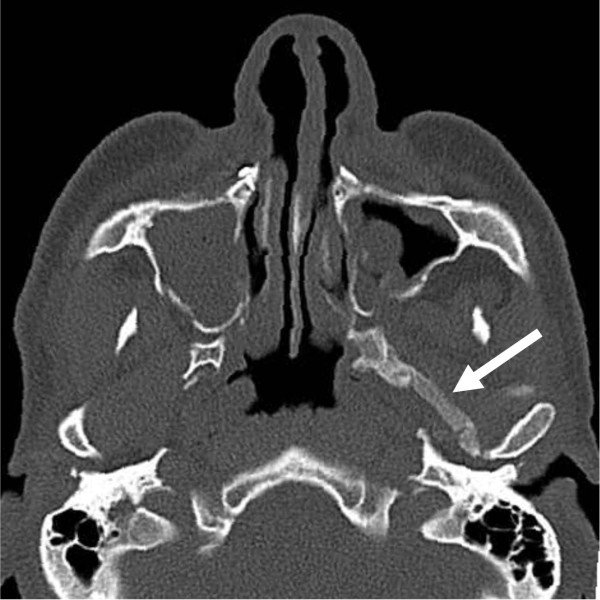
Postoperative computed tomography scan in axial projection one month after surgery demonstrating significant calcification of fibers of the left lateral pterygoid muscle (arrow).
